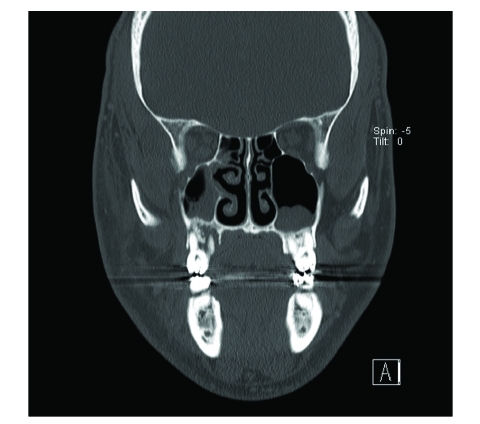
Coronal CT scan (admission) shows deterioration of right maxillary sinus floor
